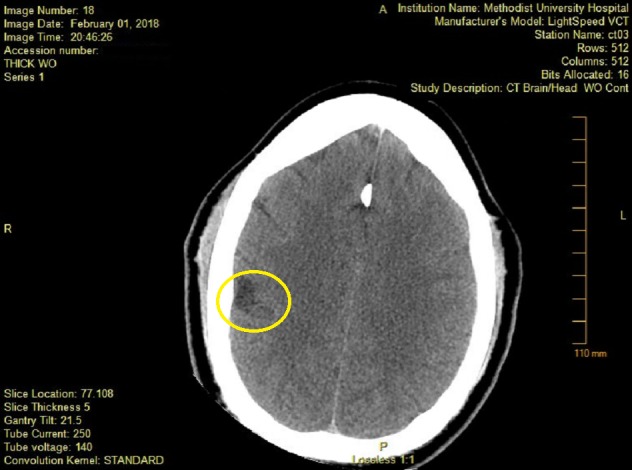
Computed tomography (CT) head on admission showing subacute right parietal cortical hypodensity. Area of interest marked by circle.
